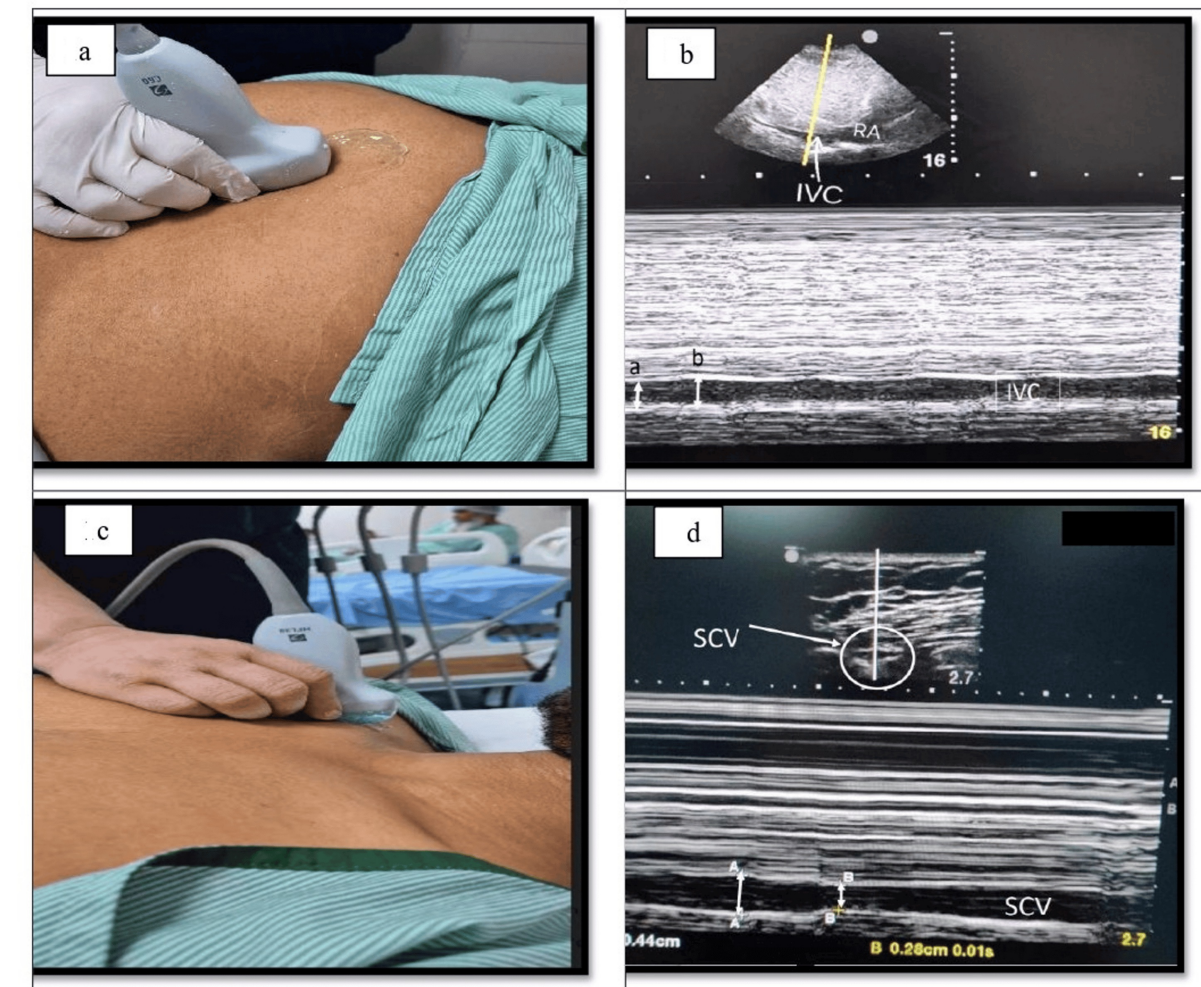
Ultrasound assessment of inferior vena cava (IVC) and subclavian vein (SCV) for dynamic diameter measurement a: Ultrasound (standard curvilinear) probe position in the subcostal region for IVC visualization. b: M-Mode tracing of the IVC, where the minimum (a) and maximum (b) diameters (marked by the double-sided white arrow) are measured perpendicular to the inner border of the vessels shown by the white line. c: Ultrasound (standard linear) probe position in the delto-pectoral groove for visualization of SCV. d: M Mode tracing of the SCV, where maximum (A) and minimum (B) diameters (marked by the double-sided white arrow) are measured perpendicular to the inner border of the vessels shown by the white line.
